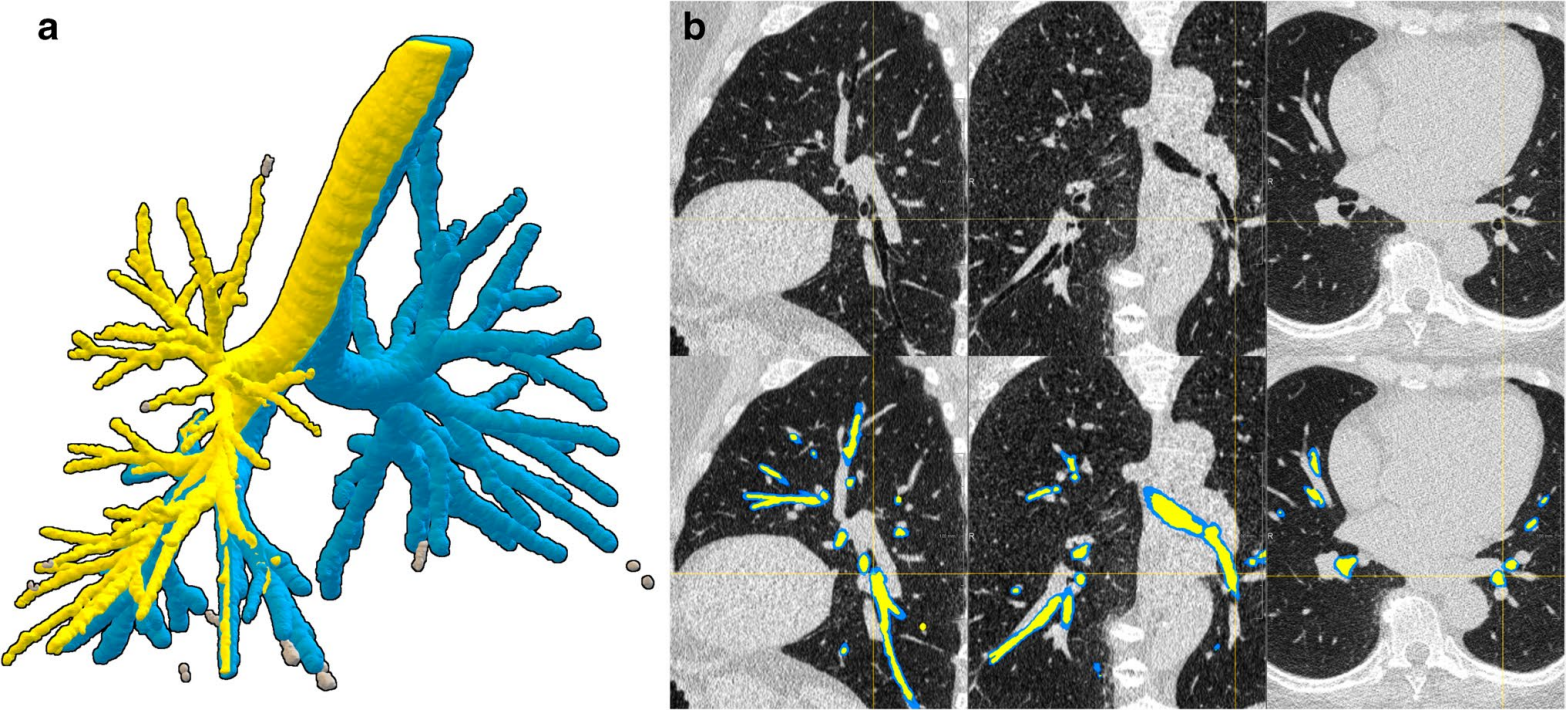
a 3D rendering of the airway lumen (yellow) and wall (blue) of an ImaLife participant CT scan. Disconnected components (grey) were discarded prior to bronchial parameter measurement. Maximum generation: 8. b 2D overlay of the airway lumen (yellow) and wall (blue) segmentations on sagittal, coronal, and axial planes respectively
